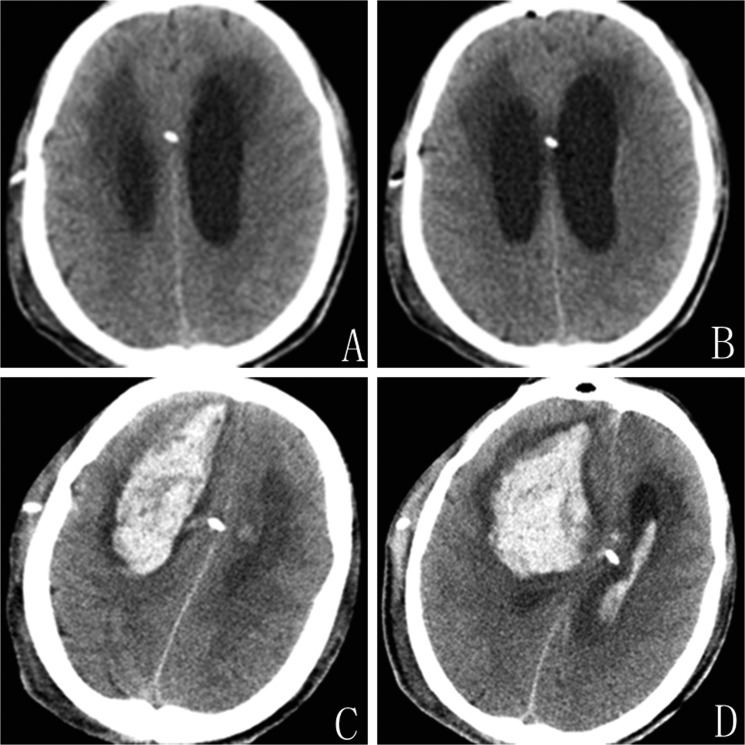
Patient 4. (A and B) CT showed no evidence of hemorrhage on postoperative day one. (C and D) CT on postoperative day four indicate large intraventricular and intracerebral hematoma along the path of ventricular catheter.
